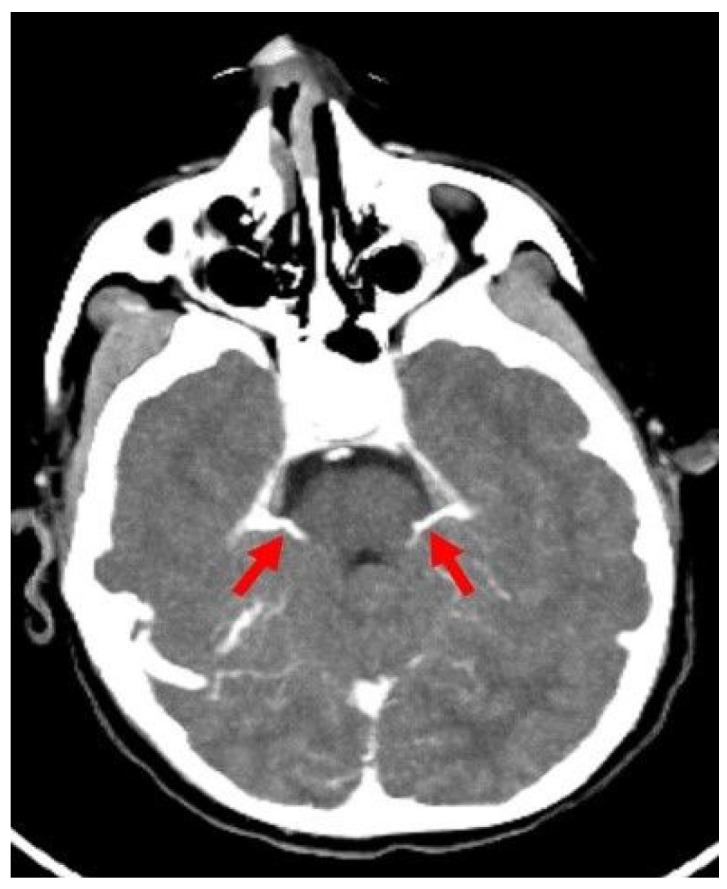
Axial CTA image of a control patient without brain death shows SPVs (red arrows) draining into the petrosal sinus at the level of the middle and superior cerebral peduncle of the mesencephalon. CTA: CT angiography, SPV: Superior petrosal vein.
